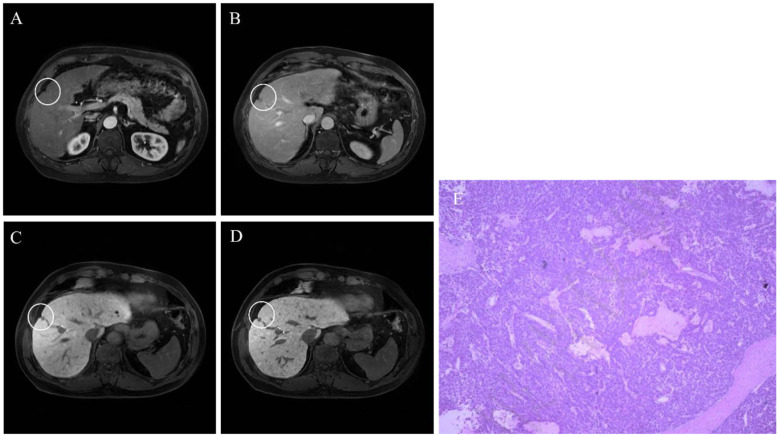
EOB-MRI demonstrated a newly detected 5 mm nodule. The lesion showed no obvious enhancement in the arterial phase (A) and exhibited rapid washout in the portal venous phase (B). Small hypointense foci were observed in 3 mm (C) and 1 mm (D) HBP. Intraoperative pathology (E) confirmed trabecular-type HCC.
